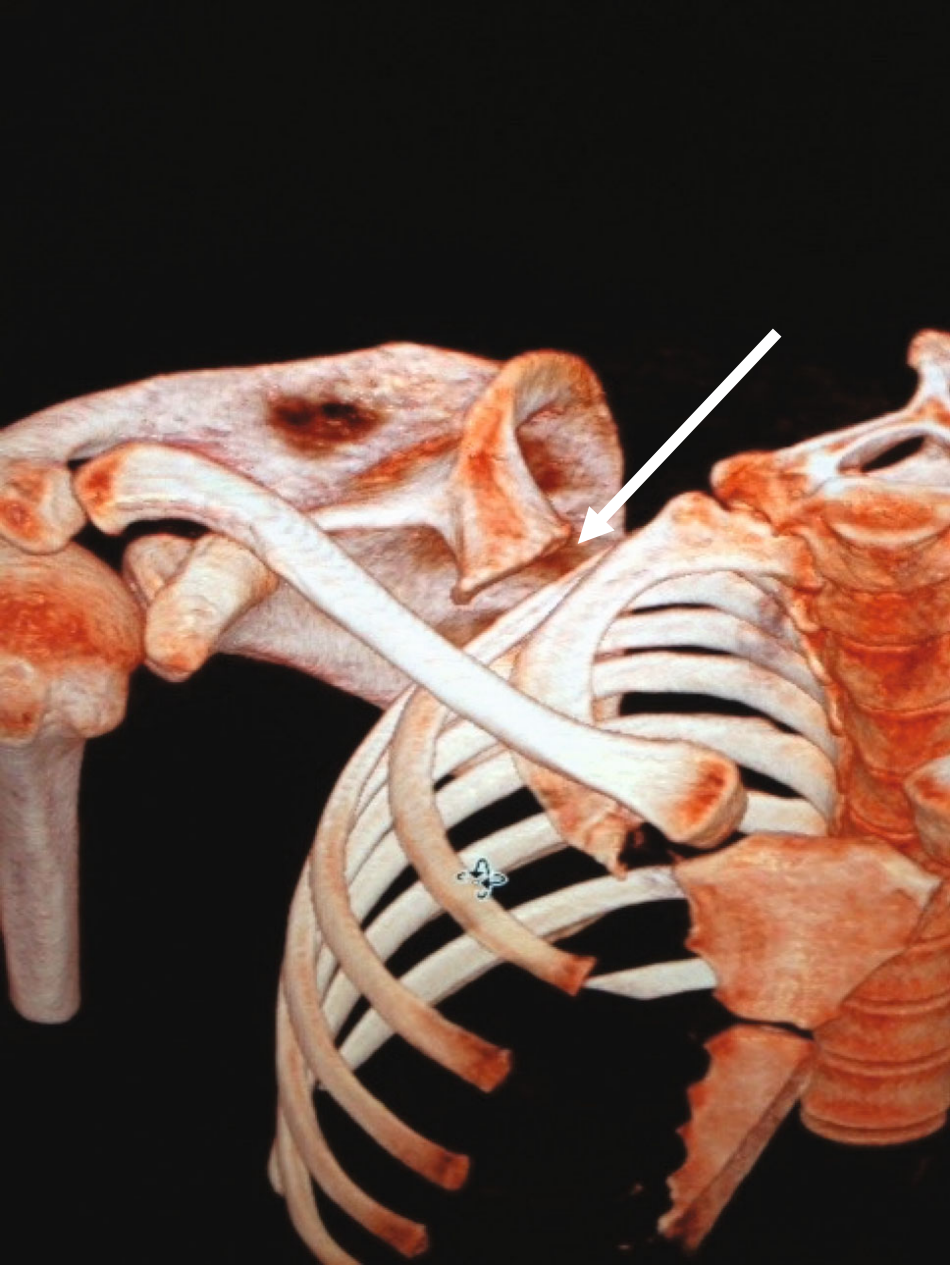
3D reconstruction CT scan of the right shoulder reveals an articulation between the second glenoid and the body of the second rib (white arrow). Reproduced from http://www.BeeMed.com, with permission.
